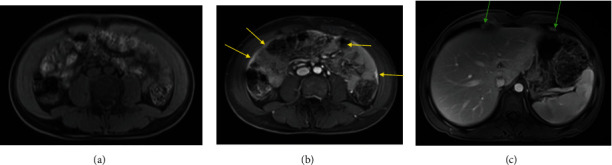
MRI of the abdomen and pelvis three months after the patient's initial MR left T1 precontrast (a), middle T1 postcontrast (b), and right T1 postcontrast (c) showed persistent but decreased peritoneal thickening/enhancement. Pulmonary nodules are decreased in size. No significant ascites is seen.
